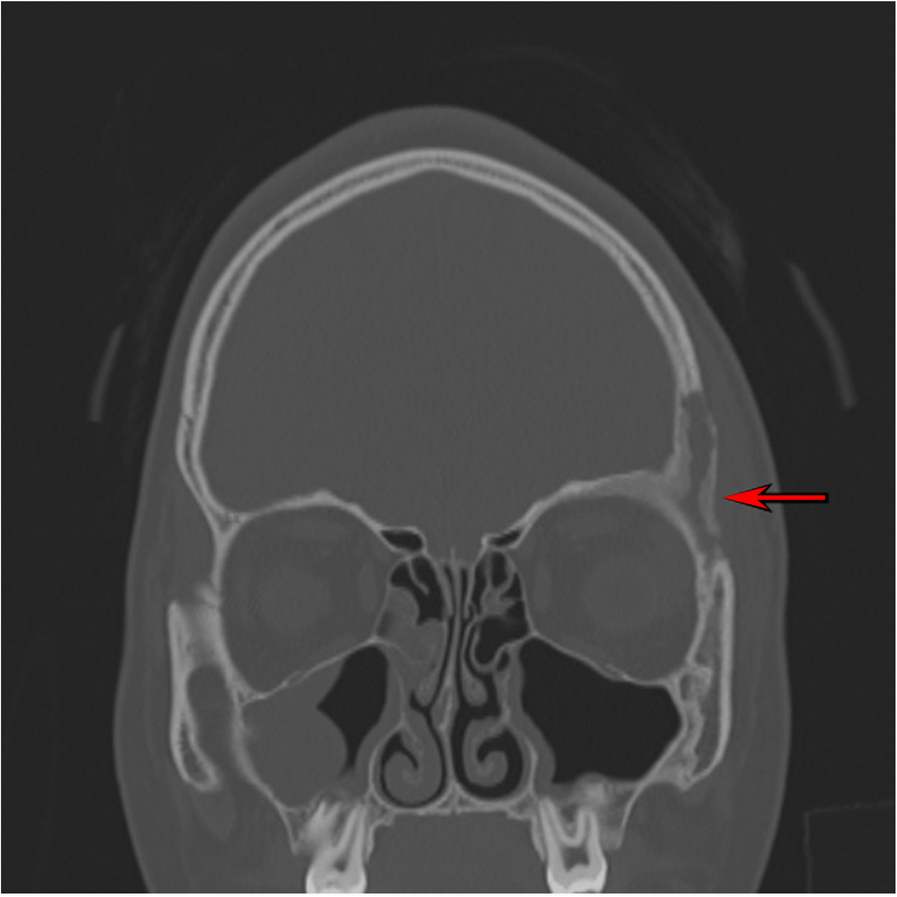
CT: remodeling and bone erosion. Note the thickness of the soft tissues (edema, inflammation)
